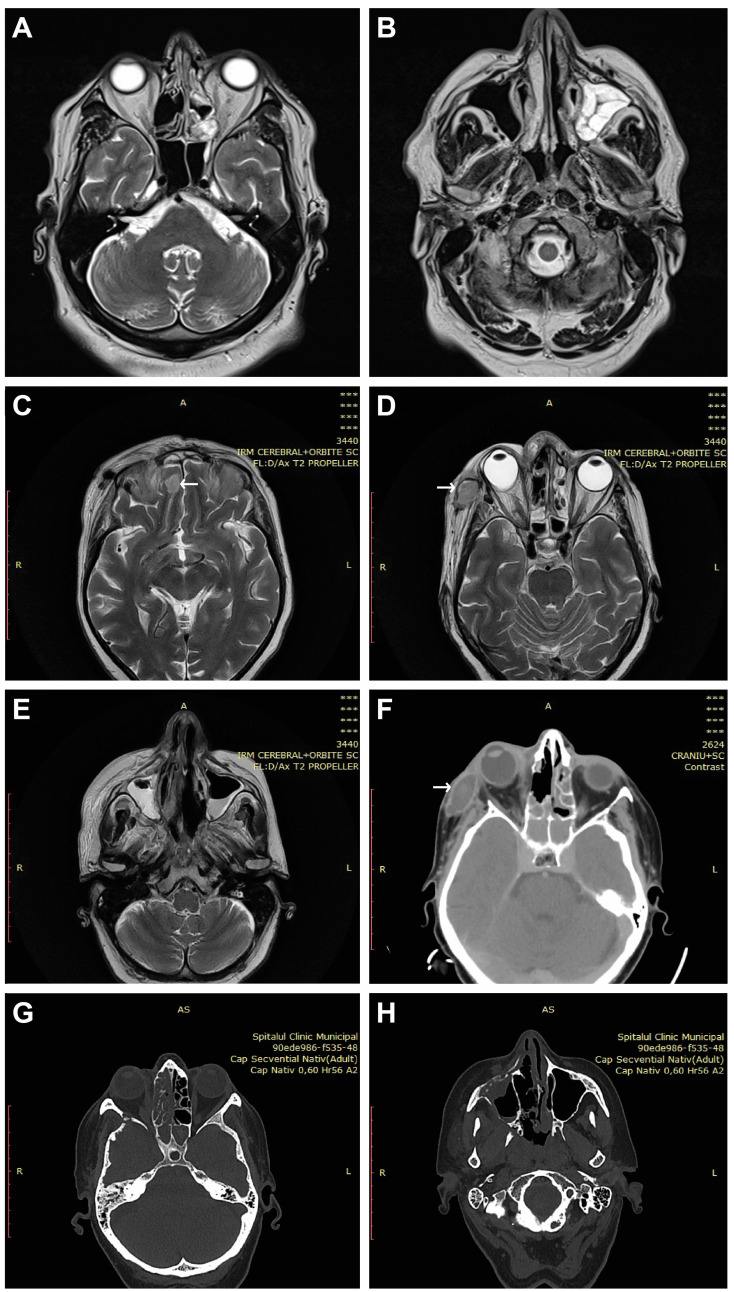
Two CT and MRI radio-imaging aspects in CAM. (A,B) Left exophthalmos and left maxillo-ethmoidal rhinosinusitis. (C) Right paramedian frontal cerebral intraparenchymal abscess (arrow). (D–F) Altered sphericity of the right eyeball, pansinusitis with inhomogeneous content, and zygomatic abscess (arrows). (G,H) Right pansinusitis and bone erosions.
